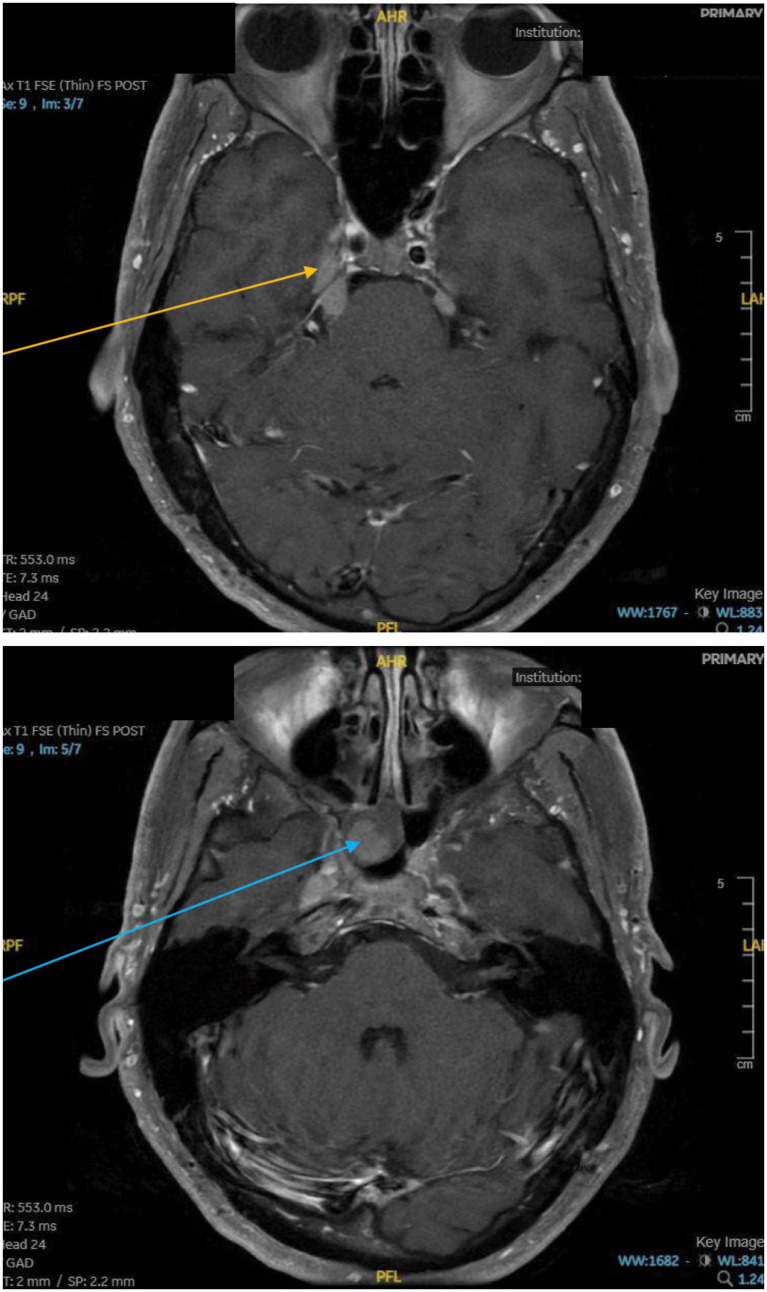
Axial MRI of Internal Auditory Canal showing enhancement within the right Meckel’s cave (Orange arrow), and Right Cranial Nerve V3 segment (Blue arrow).
